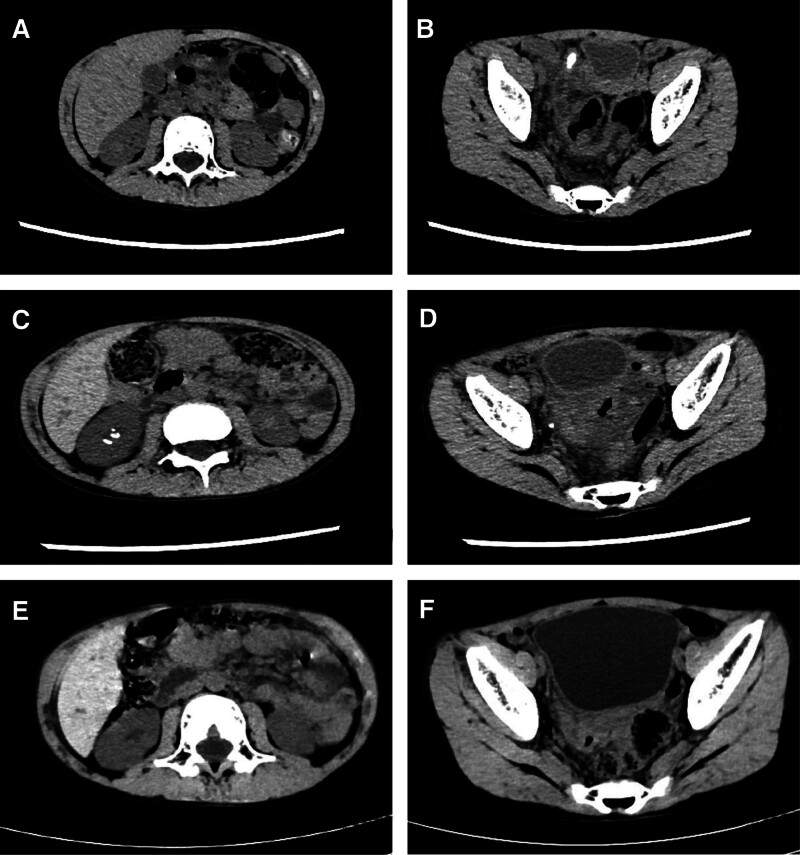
CT features during calculus treatment in the child: CT scan before treatment with ceftazidime showing no kidney stones (A) and no ureteral stones (B); CT scan 1 week after treatment with ceftazidme showing kidney stones (C) and ureteral stones (D); no kidney stones (E) or ureteral calculi (F) were detected by CT after treatment. CT = computed tomography.
